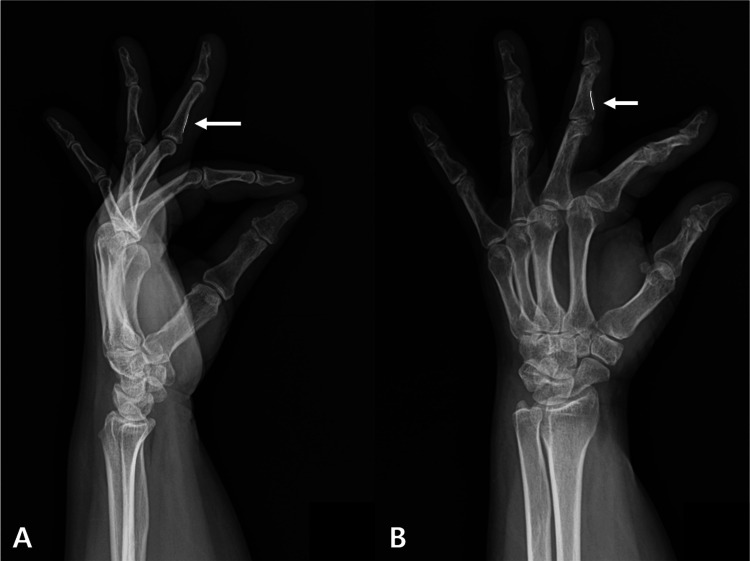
In lateral (A) and oblique (B) X-rays, a bony ridge (white arrow) is observed outside the normal line (white line) of the middle phalanx.
